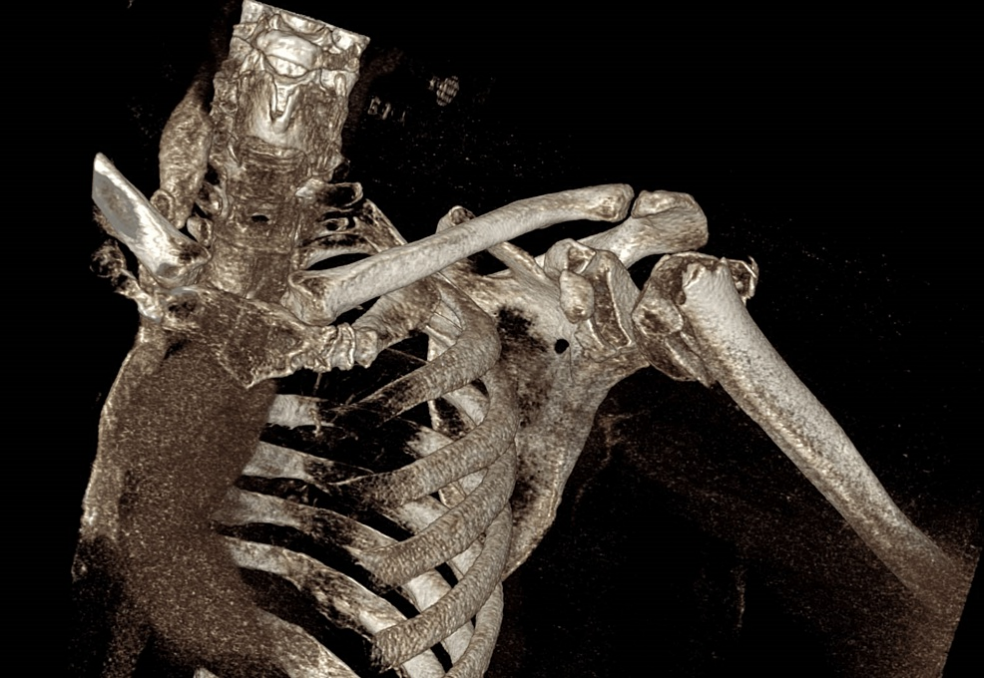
CT reconstruction demonstrating the entire injury pattern without subtraction of other bony structures
